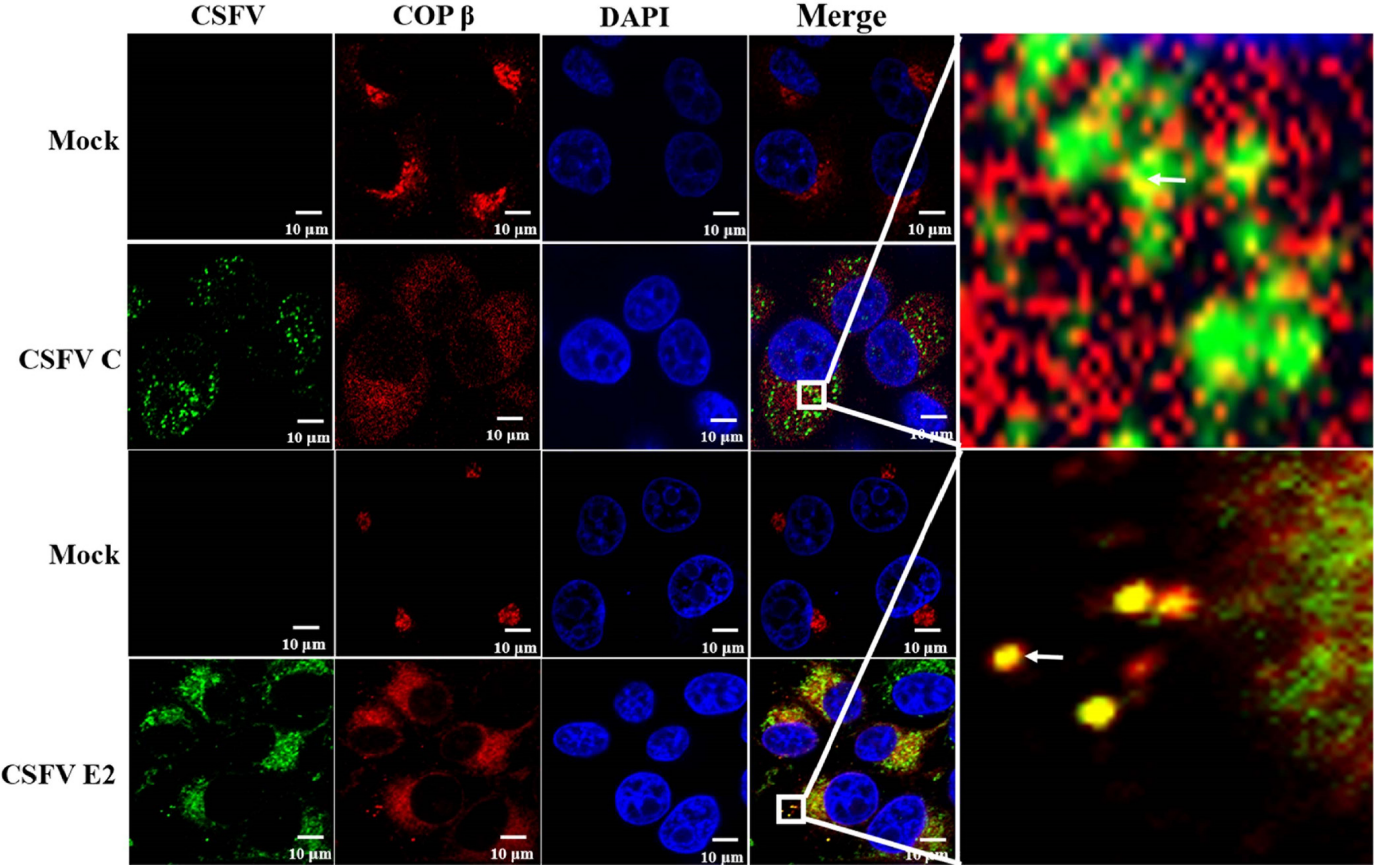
CSFV core and E2 proteins colocalized with COP I. SUVECs were infected with CSFV (MOI of 1) for 12 h and fixed in 4% paraformaldehyde. Next, cells were costained with anti-E2 and anti-COPβ antibodies or costained with anticore and anti-COPβ antibodies.
